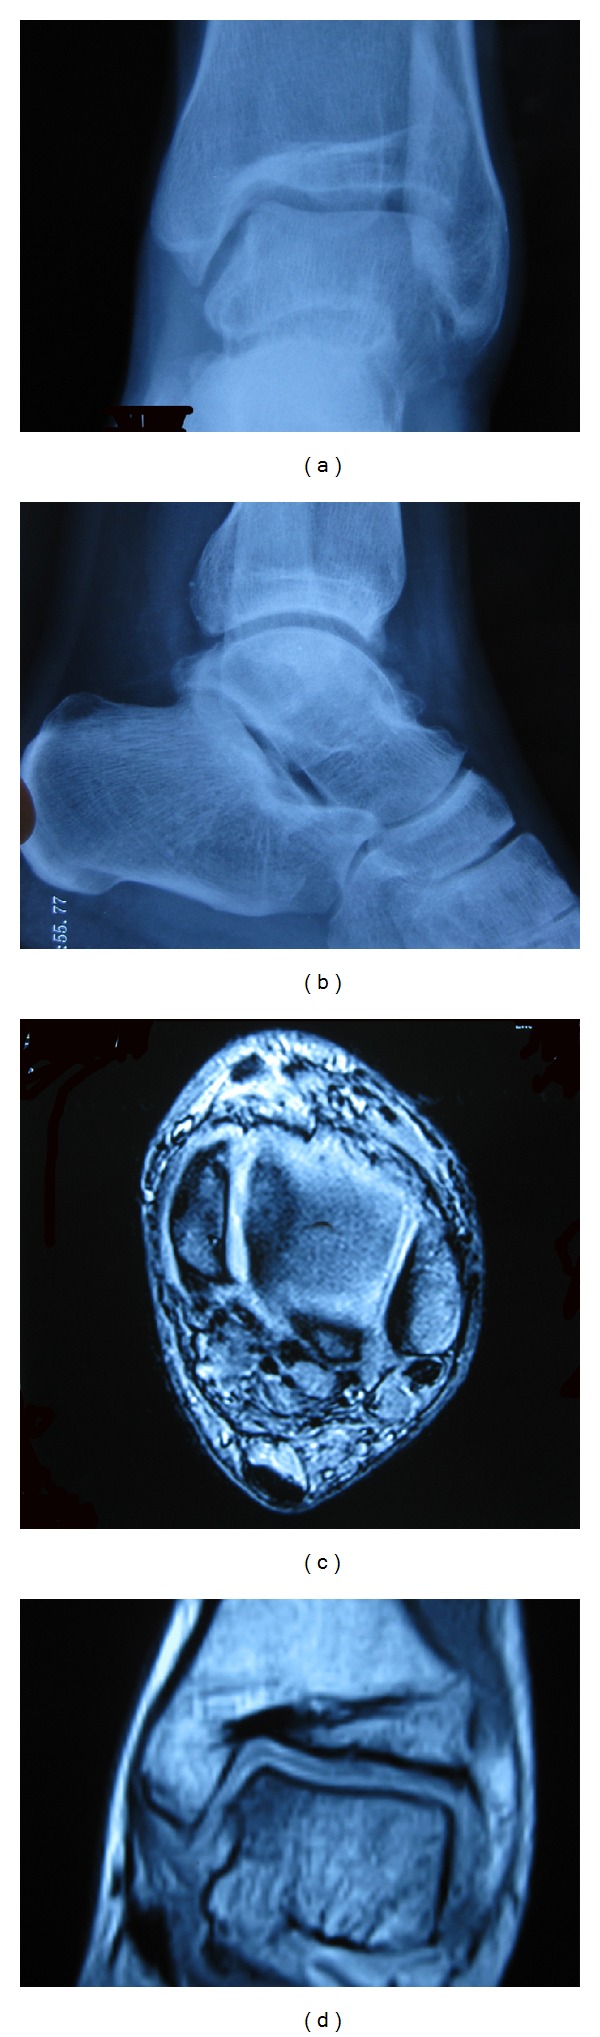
Anterolateral (a) and lateral (b) radiographs of the patient's left ankle before the ankle arthroscopy showed preservation of the joint space with mild anterior osteophytes. (c, d) magnetic resonance imaging showed the presence of osteochondral lesion of the medial talar dome.
